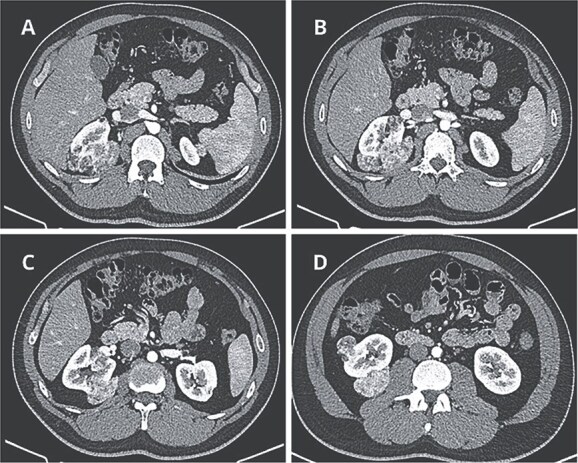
Preoperative CT scan. (A) Axial CT scan showing AML surrounding the right diaphragmatic crus. (B–D) Lesions composed of fat and soft-tissue densities in the upper, middle, and lower poles of the right kidney extending into adjacent retroperitoneal structures.
